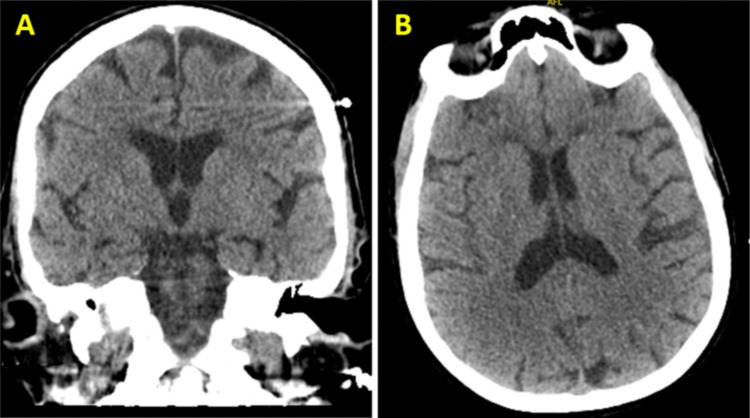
Computed tomography (CT) scan of the brain without intravenous contrast. (A) The coronal and (B) axial brain scans reveal no signs of acute intracranial pathology. This includes a normal density of the brain parenchyma, the absence of acute intracranial hemorrhage, and no indications of abnormal masses, growths, mass effect, midline shift, or hydrocephalus.
